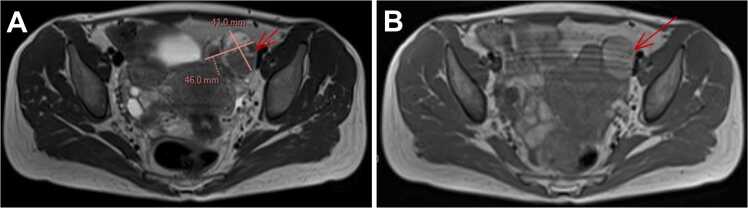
Pelvic magnetic resonance imaging. (A) Axial T2 sequence showing a multiloculated left ovarian cystic mass (41 × 46 mm) with a fluid-fluid level. (B) Axial T1 sequence showing intracystic hyperintensity without suspicious enhancement, consistent with proteinaceous or hemorrhagic content.
